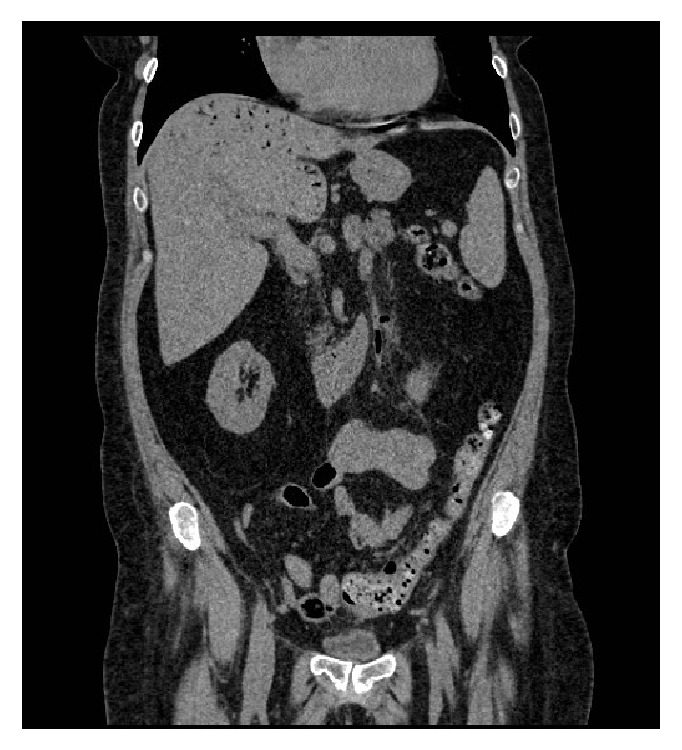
CT abdomen/pelvis of patient 3. Representative CT showing numerous tubular structures within the liver filled with gas extending to the subcapsular liver surface, felt to be secondary to a perforated diverticulum in the sigmoid colon and secondary abscess.
